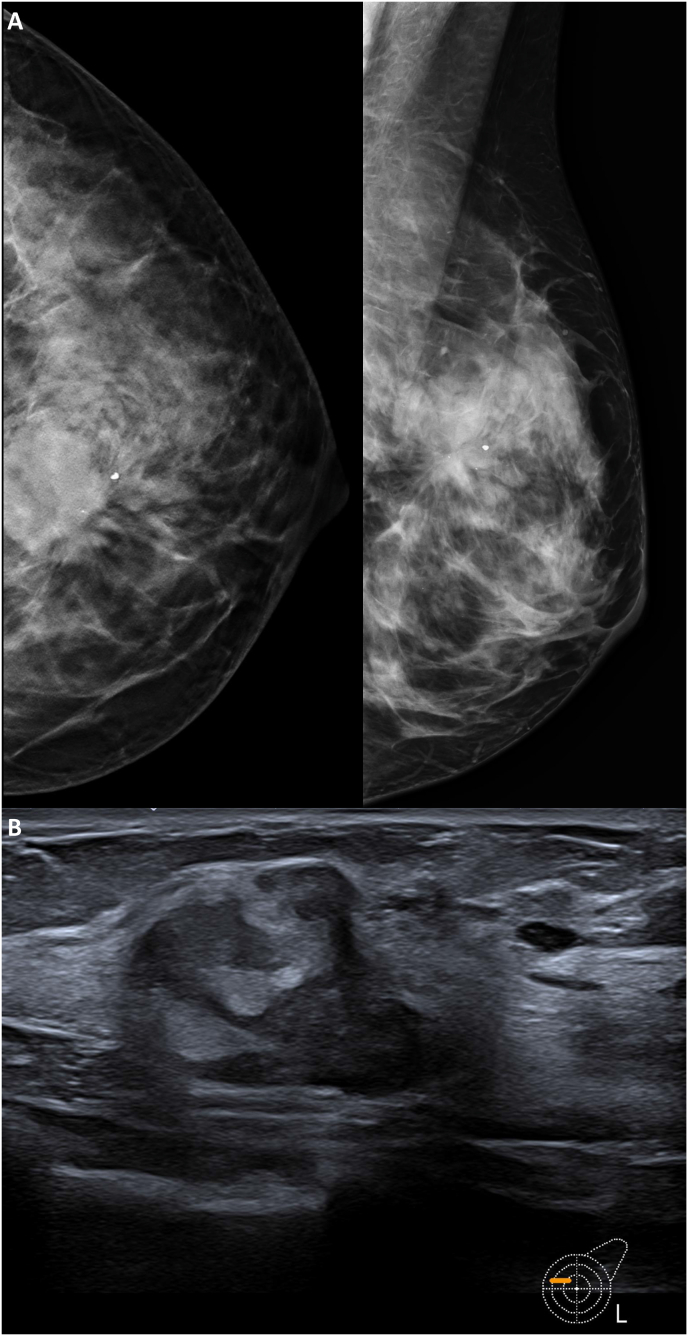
Radiology assessments obtained at diagnosis Legend. A: DBT of left breast (CC and MLO views) shows a irregular mass with spiculated margins and calcifications associated in the upper inner quadrant of left breast. B: Breast US shows a irregular and heterogeneous mass. DBT: digital breast tomography; CC: craniocaudal; MLO: mediolateral oblique; US: ultrasound.
